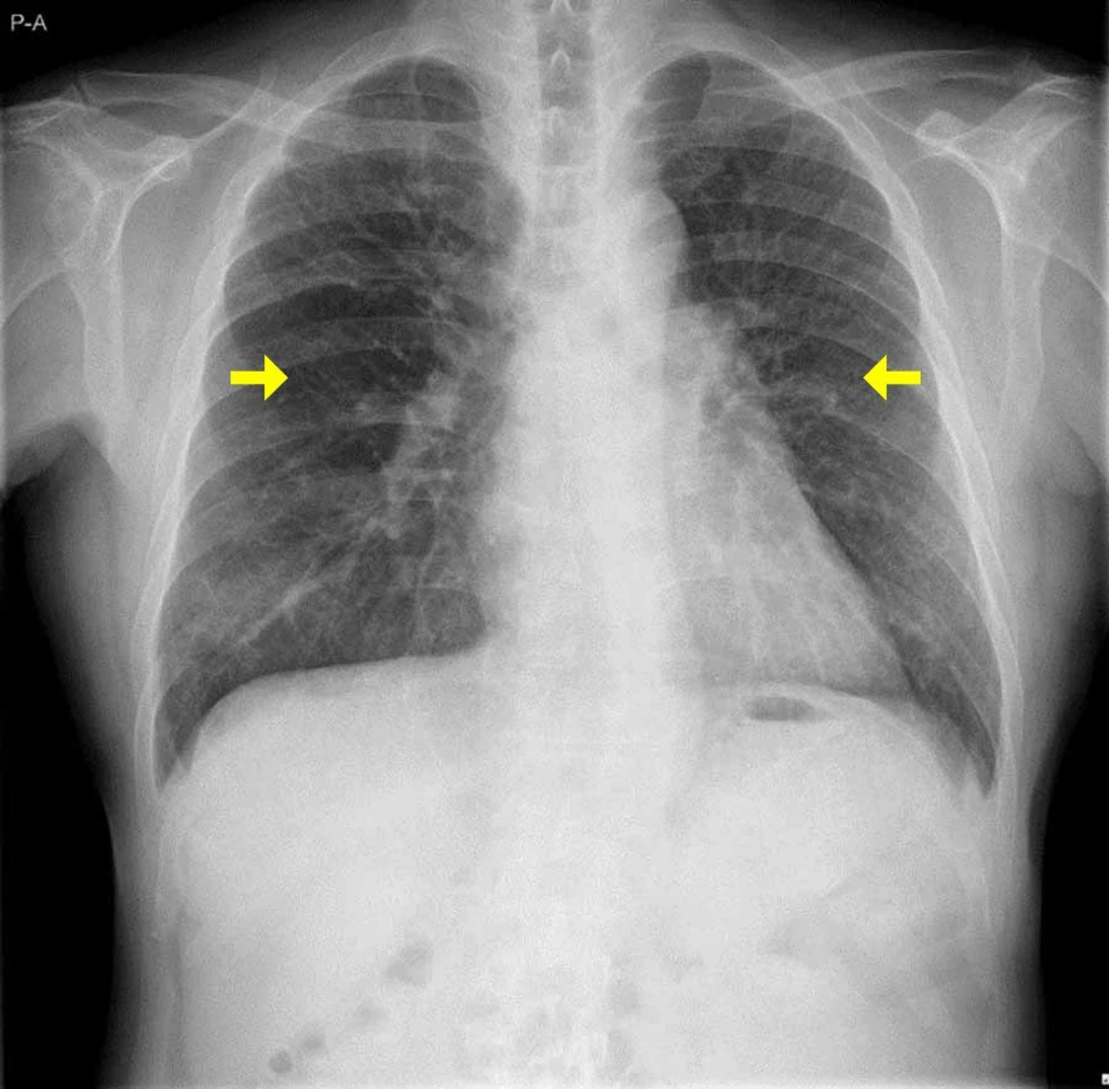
Chest X-ray before discharge from the hospital Chest X-ray showing normal lung field before discharge from the hospital.
